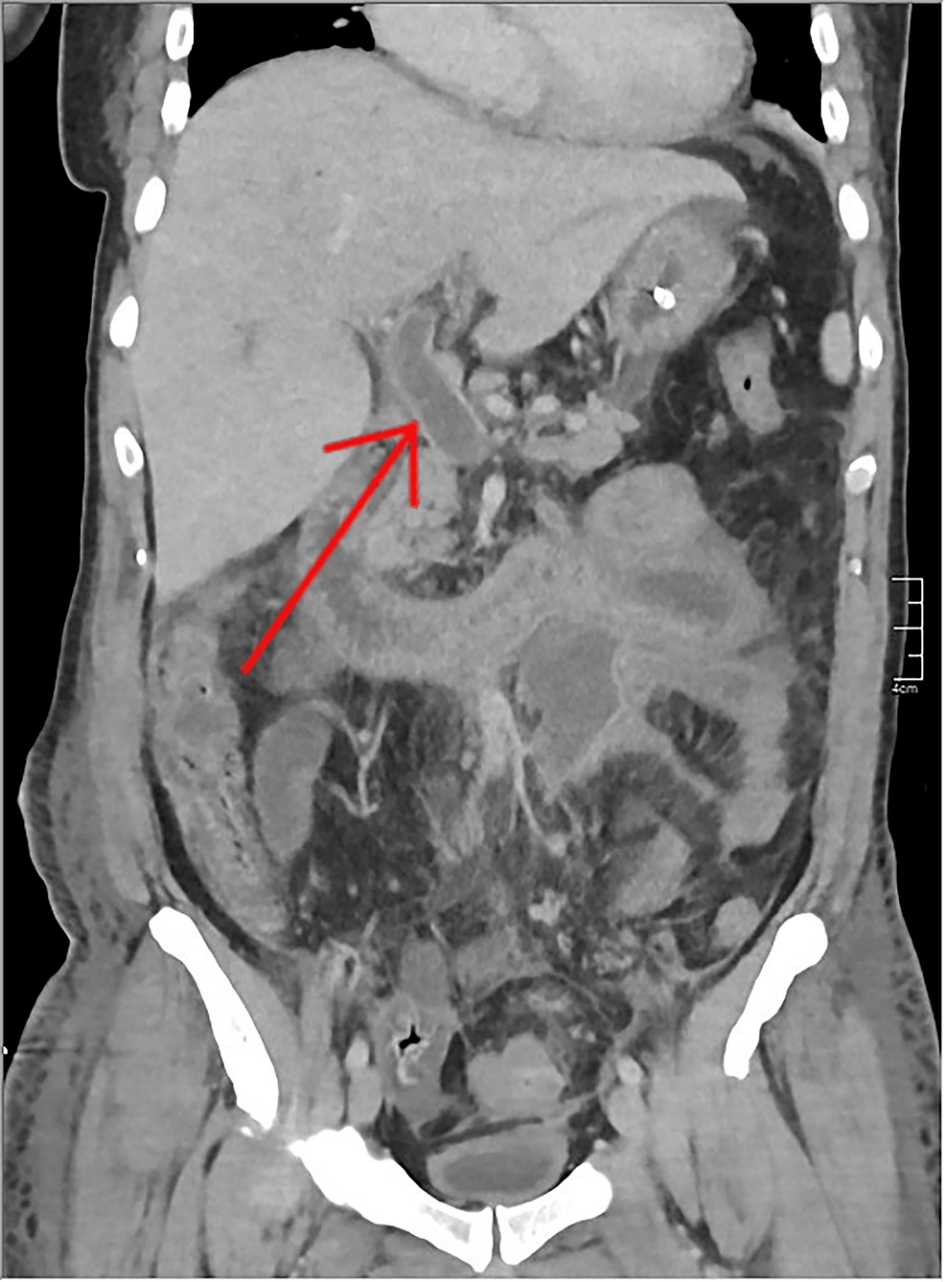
Abdominal CT scan in the portal phase showing portal vein thrombosis. CT, computed tomography
